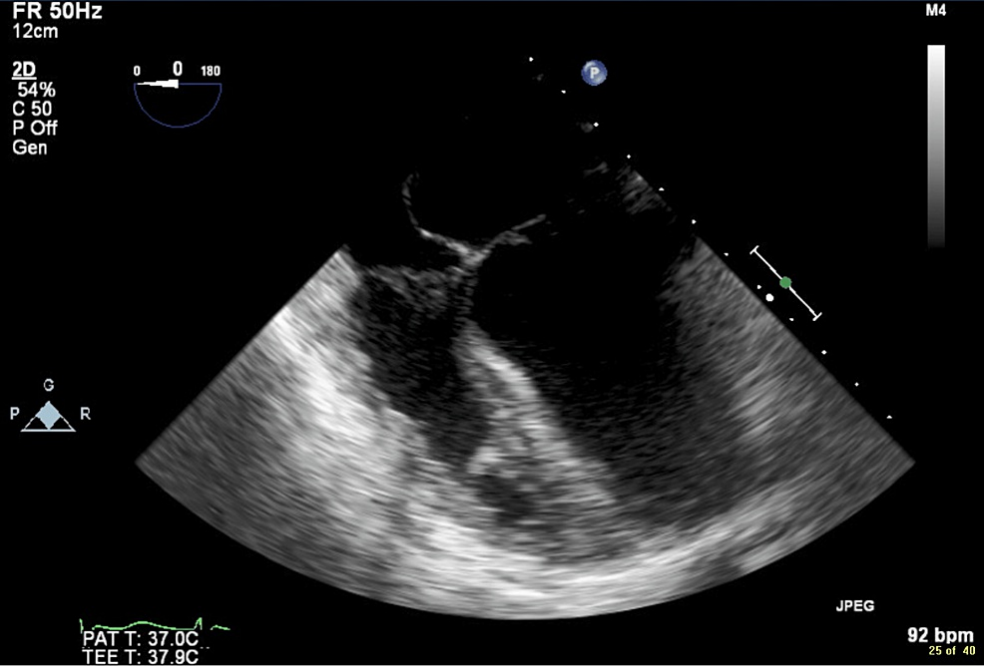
Mildly dilated left ventricle on transesophageal echocardiogram; the interventricular septum was slightly hypokinetic on motion capture.
